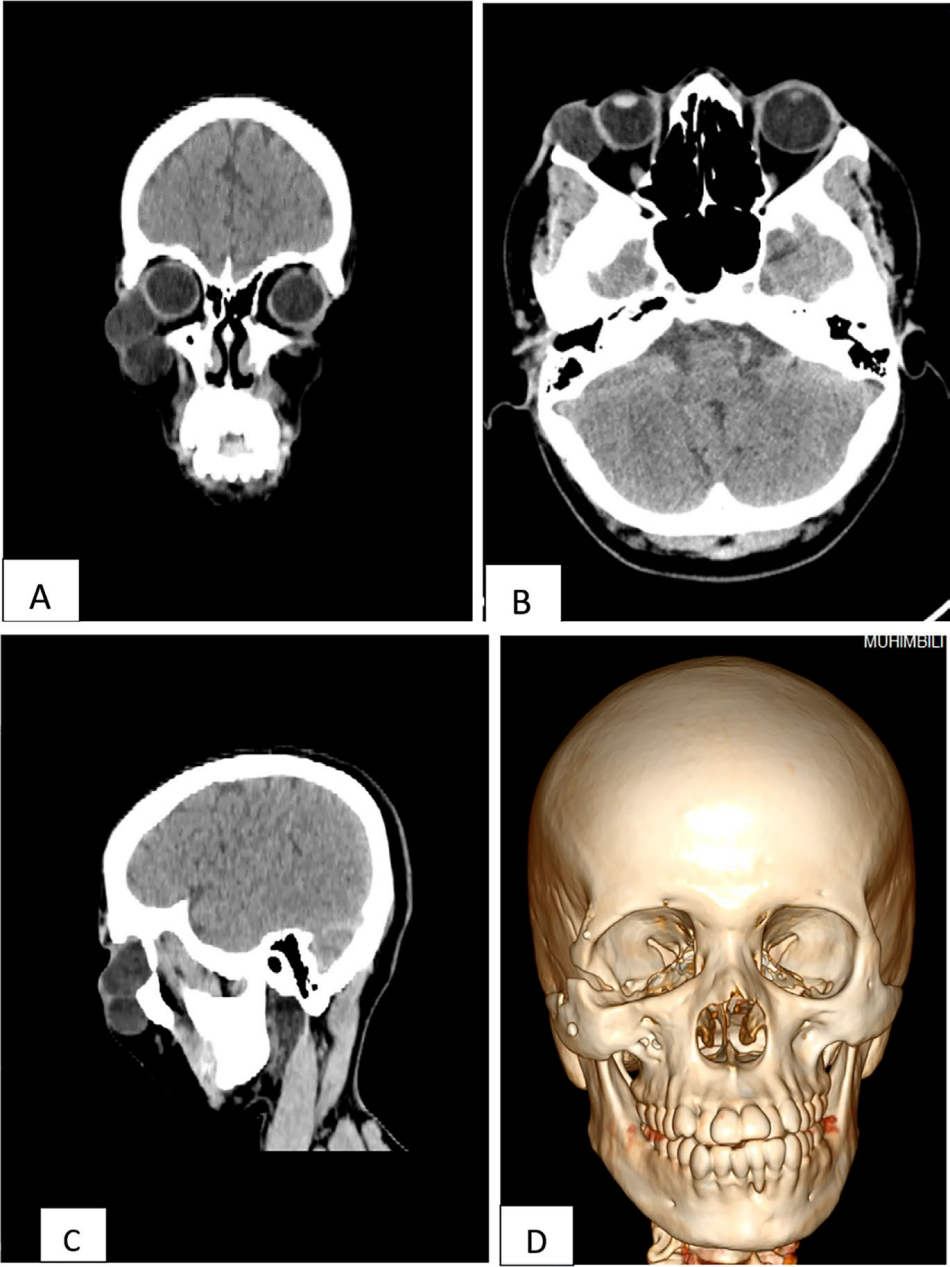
CT scan views (A–C), coronal, axial, sagittal cuts showing the cysts on the orbit and infraorbital regions. (D) A 3D reconstruction CT showing the non‐union lateral orbital rim fracture, see the increased orbital volume on the right orbit signifying a displaced zygomaticomaxillary fracture.
